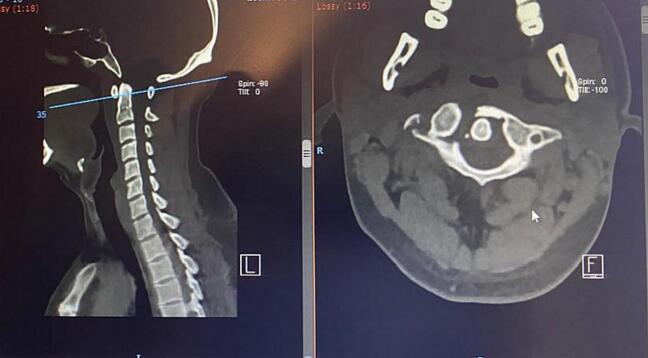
CT axial of the cervical spine. Axial CT showed complete fracture of the right posterior and anterior arch of C1 and disruptions.
